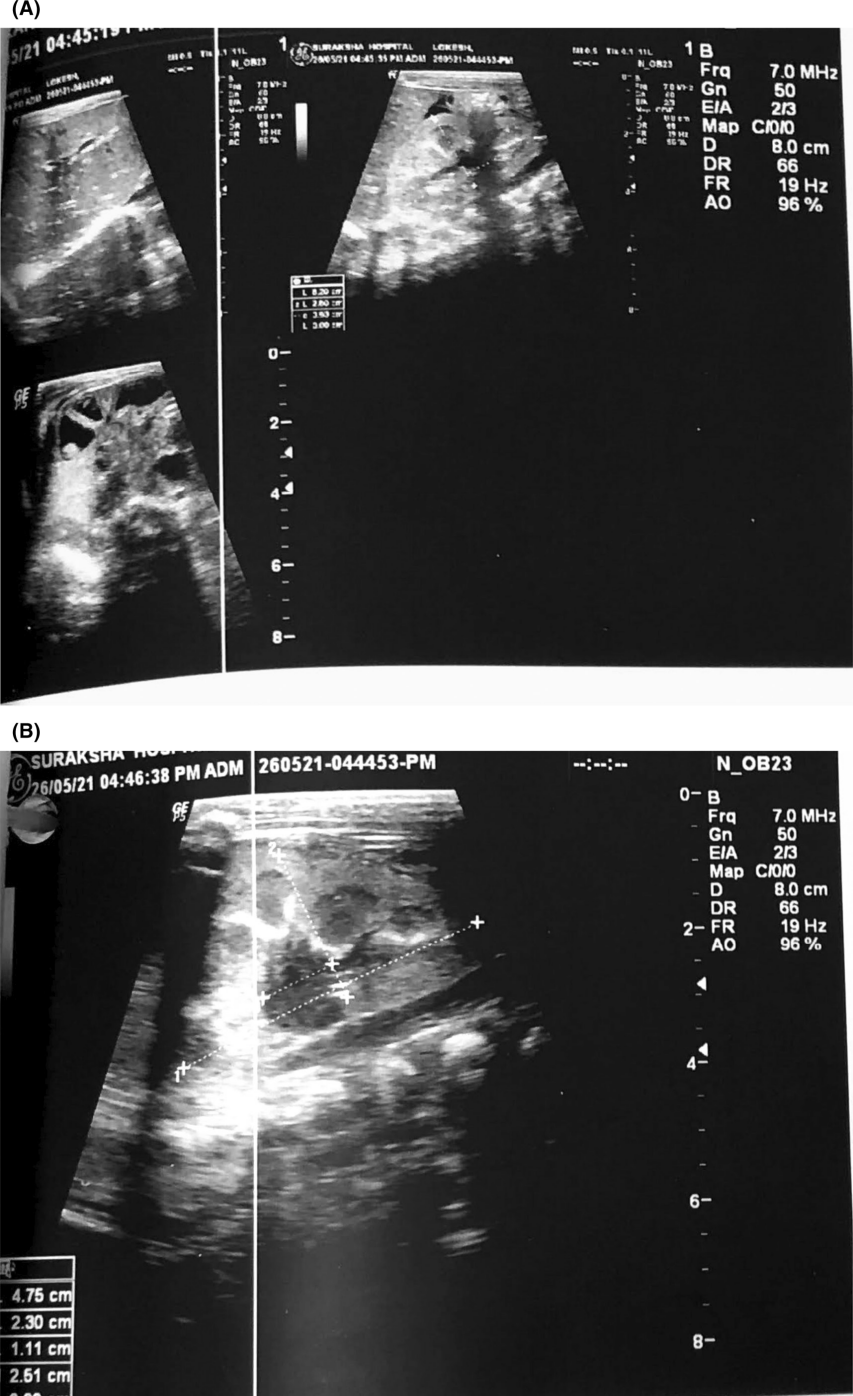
(A) USG showing bilateral increased renal echotexture. (B) Ultrasound showing a prominent left renal pelvicalyceal system with an 11 mm AP diameter of the renal pelvis
